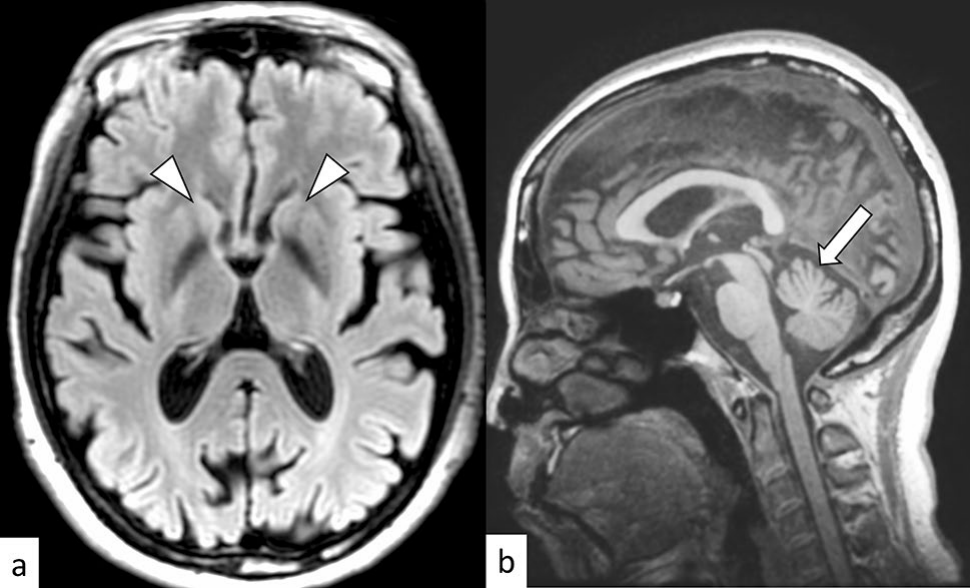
A 40-year-old woman with SCA17. FLAIR axial image shows atrophy of the bilateral caudate nuclei (a, arrowheads). T1-weighted sagittal image b shows cerebellar atrophy (arrows), while the brainstem is spared
